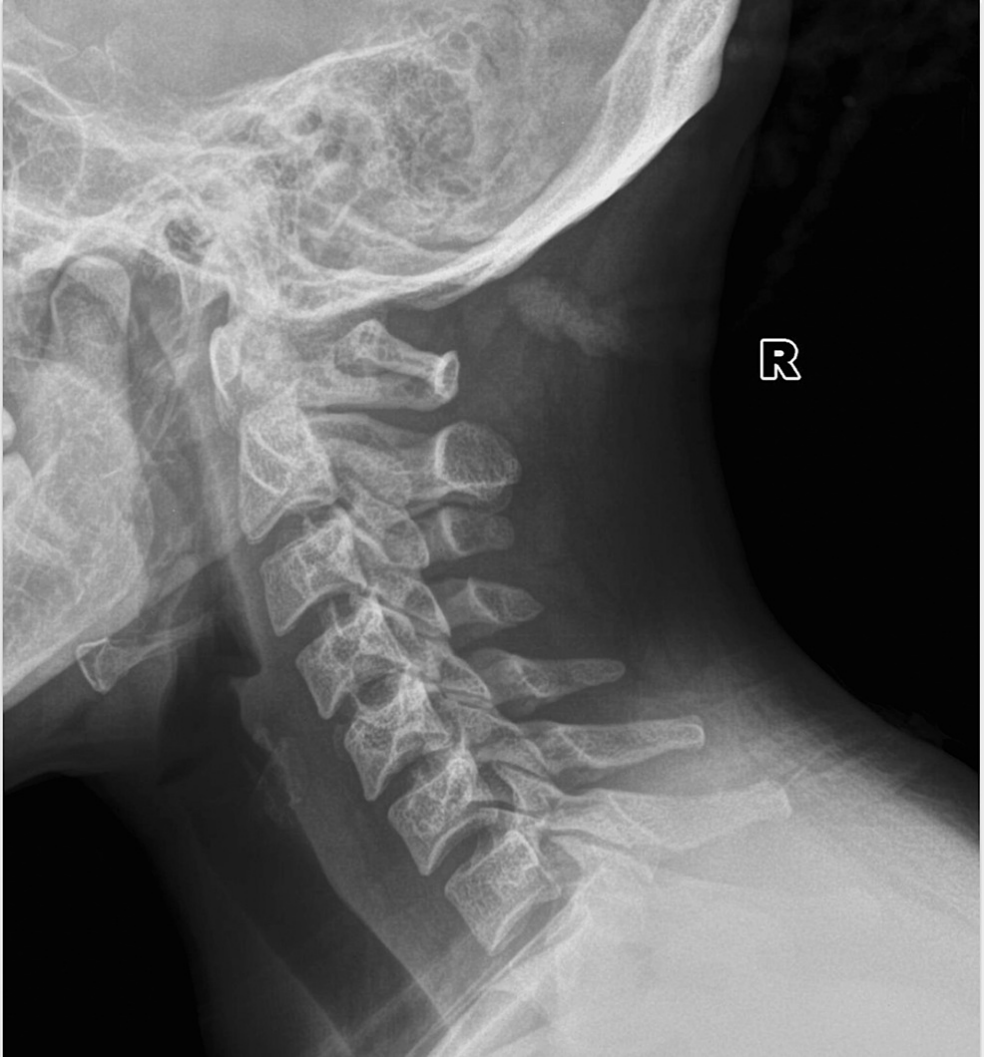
Sample X-ray image of a normal spine
